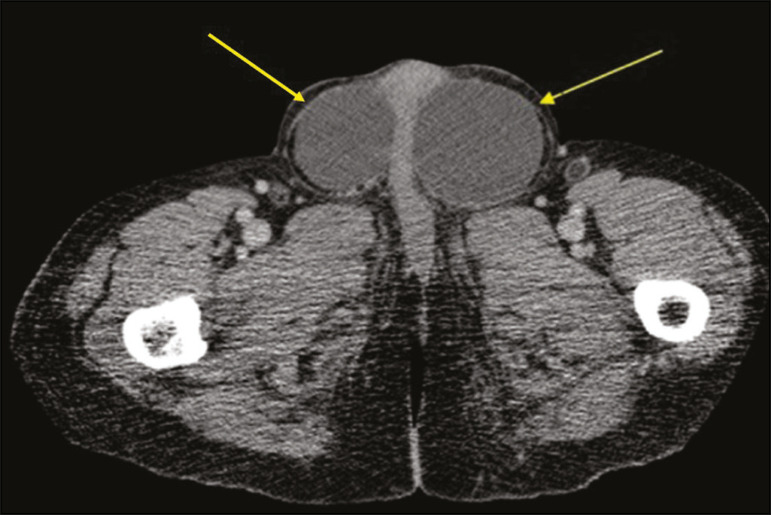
Unenhanced axial CT in a patient with cirrhosis, showing ascites and large inguinoscrotal hernias containing ascitic fluid.
